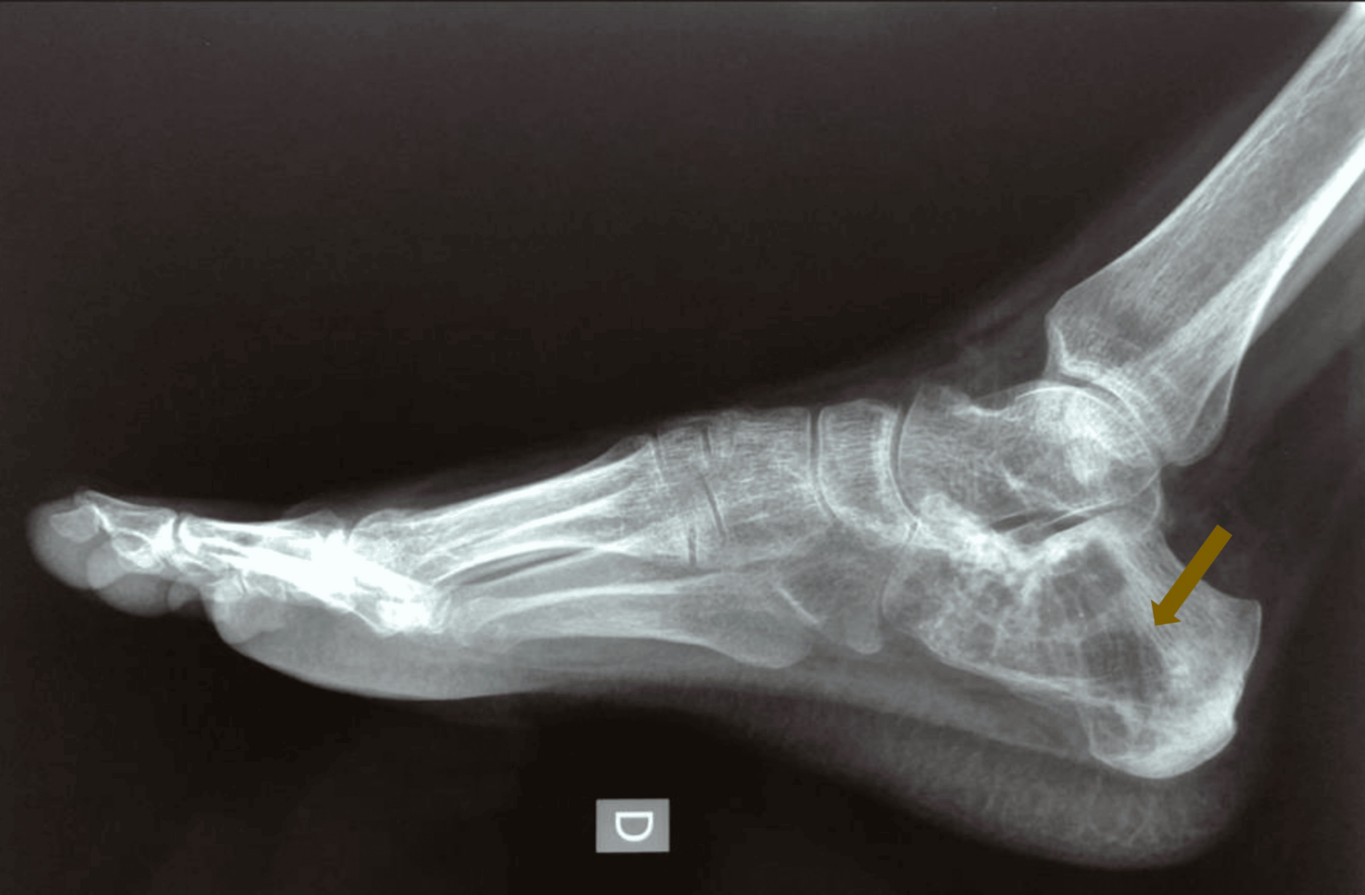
Plain radiograph of the right heel showing a well defined, expansile lytic lesion in the calcaneus. Well-defined, expansile lytic lesion in the calcaneus consistent with aggressive bone tumor; absence of periosteal reaction suggests a primary malignant lesion rather than infection.
